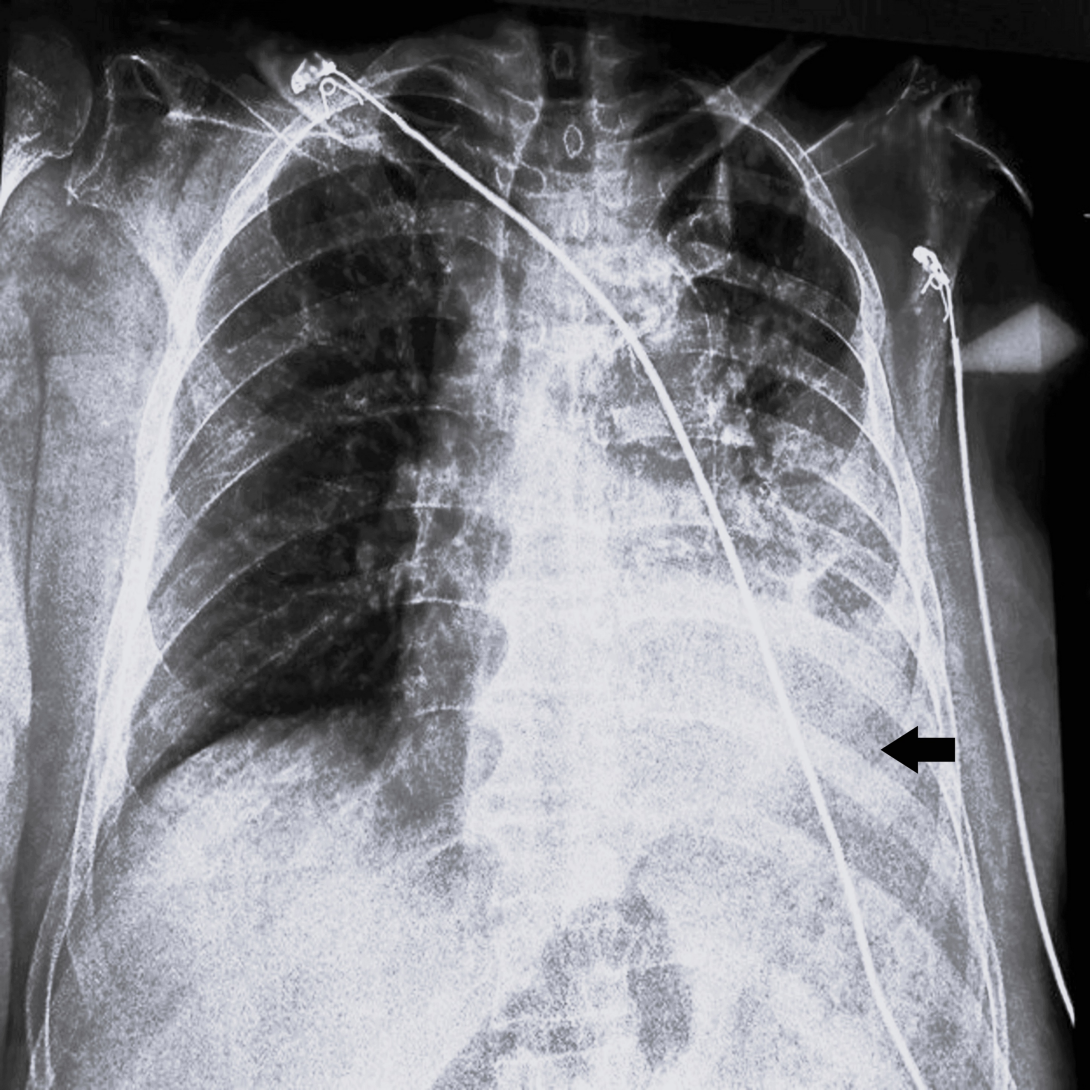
Chest X-ray (posteroanterior view) suggestive of left-sided lobar pneumonia (black arrow pointing toward the segments of consolidation).
